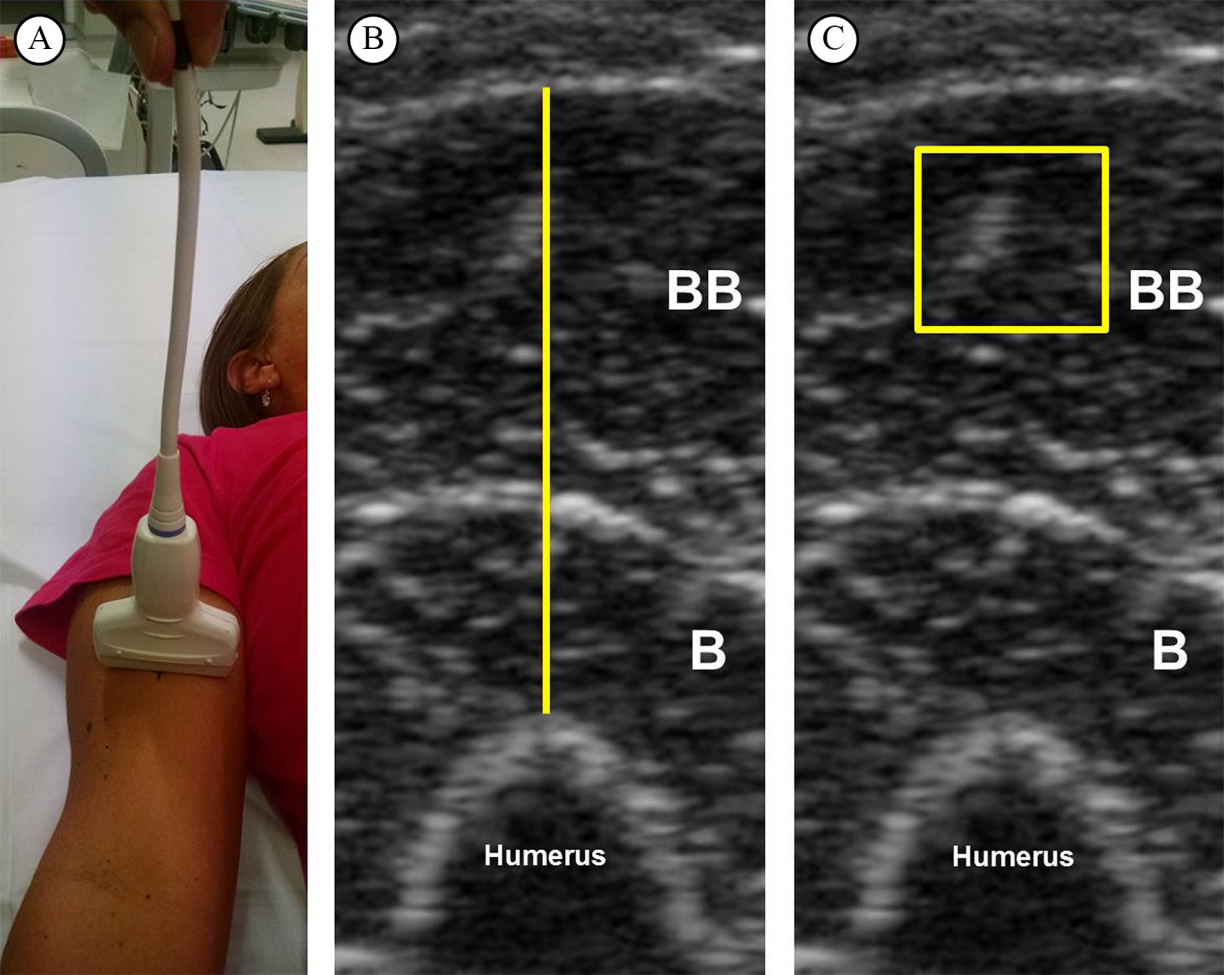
Representative image from one subject. (A) Ultrasound scan of forearm flexor muscles. (B) Muscle thickness of forearm flexors, defined as the distance between adipose tissue and bone. (C) Echo intensity of biceps brachii muscle in a square set at 100 mm^2^. BB: biceps brachii; B: brachialis.
